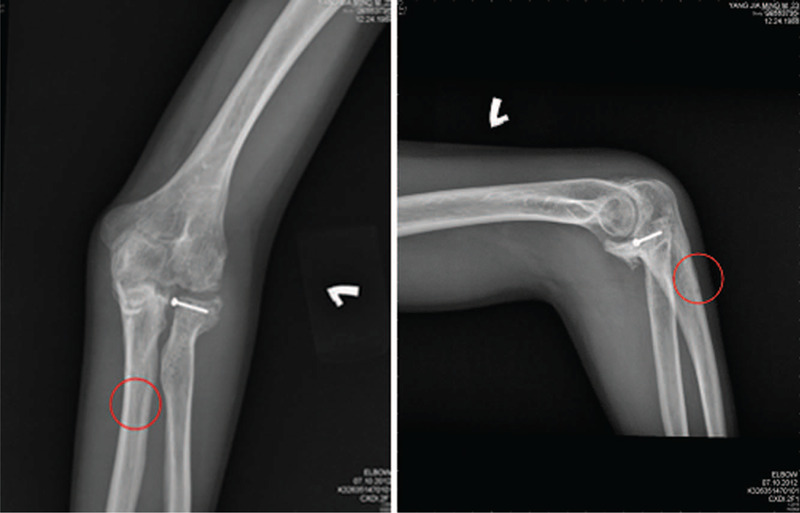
The post removal of the internal fixation X-ray showed the bone union of the fracture site and smooth cortical bone without obvious fracture around the previous fracture site or the drill holes. The drill hole (the red circle) is visible after removal of the screw.
